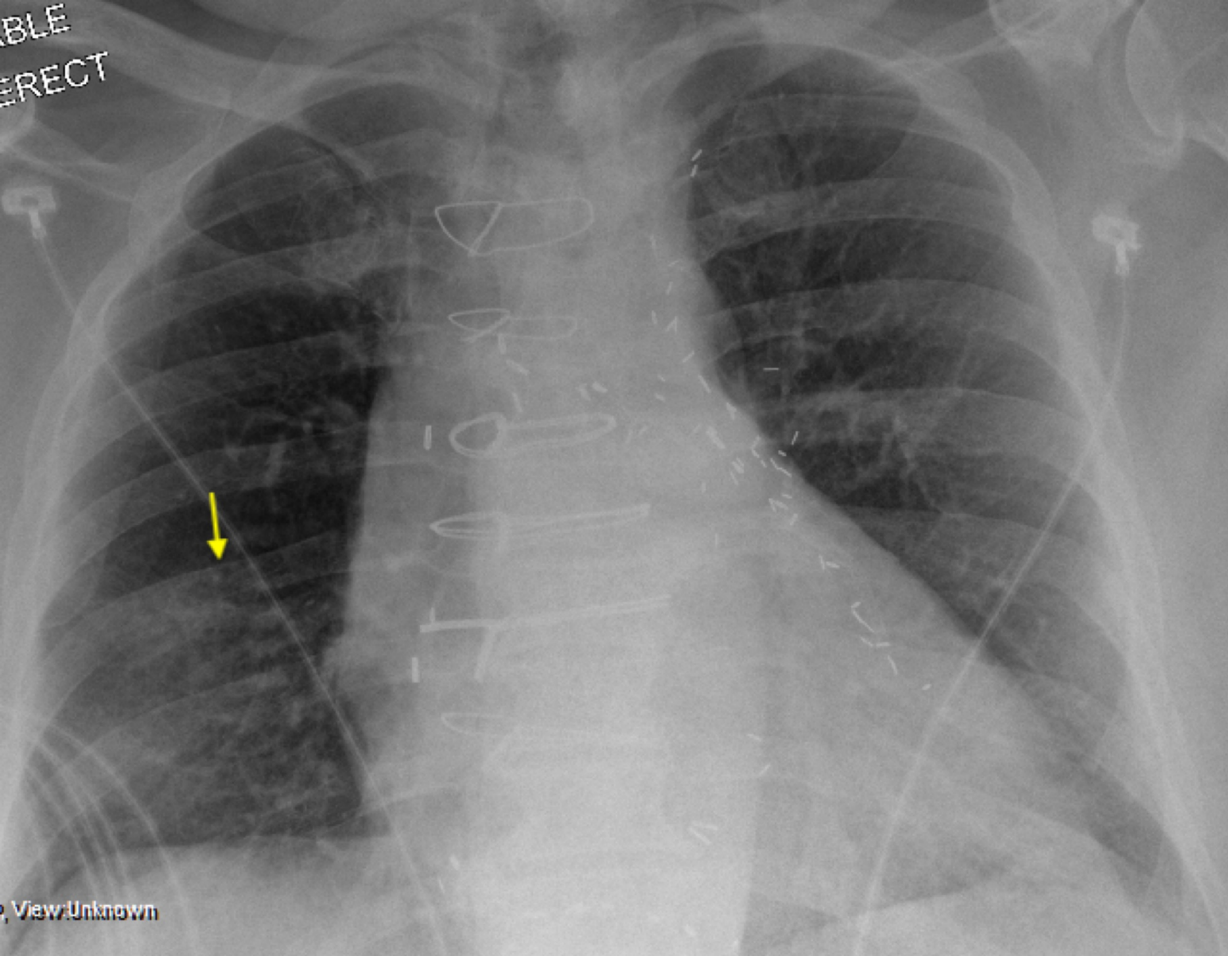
Chest Radiography of a Patient with Mild COVID-19 Chest radiography on admission showed patch infiltrate on right lower lobe.
